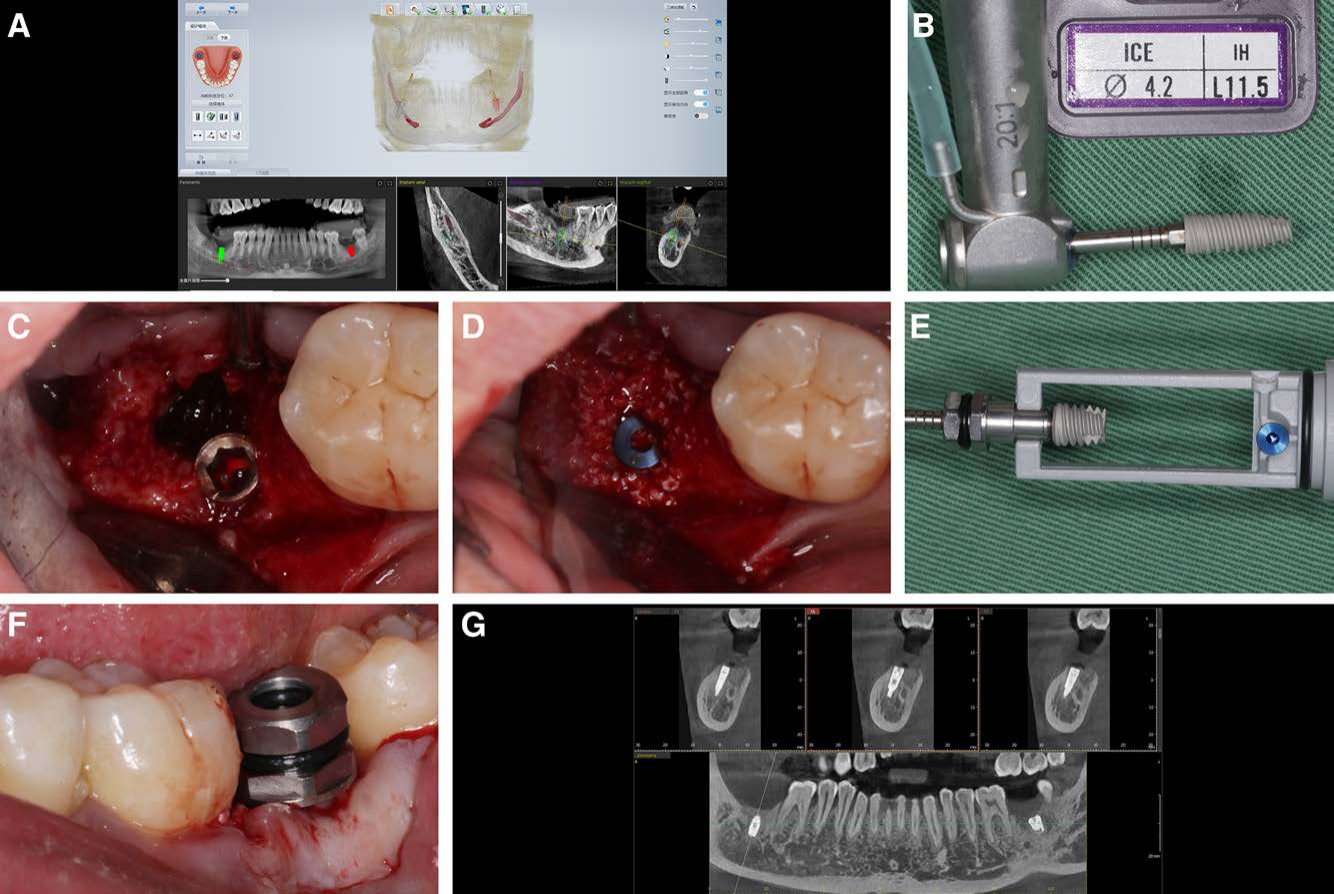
(A) Preoperative planning using the dynamic navigation system (DCARER, China); (B) Alpha-Bio Tec, ICE, ABT1021 implant (diameter 4.2 mm, length 11.5 mm); (C) implant placement at site #46; (D) filling the gap between the implant and bone wall with Bio-Oss® (Geistlich, Switzerland) bone graft material; (E) Alpha-Bio Tec, ICE, ABT1021 implant (diameter 4.2 mm, length 11.5 mm); (F) Alpha-Bio Tec, ATID implant (diameter 5 mm, length 6 mm); (G) immediate postoperative CBCT.
